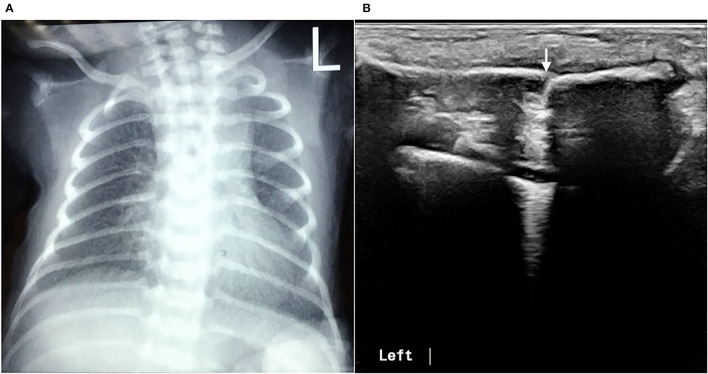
Missed clavicle fracture on X-ray. The infant underwent a chest X-ray due to postnatal dyspnea, but there were no signs of fractures in either clavicle (A). Physical examination found a bone rubbing sensation on the left clavicle on admission, and the subsequent ultrasound examination confirmed the presence of a fracture of the left clavicle (B).
